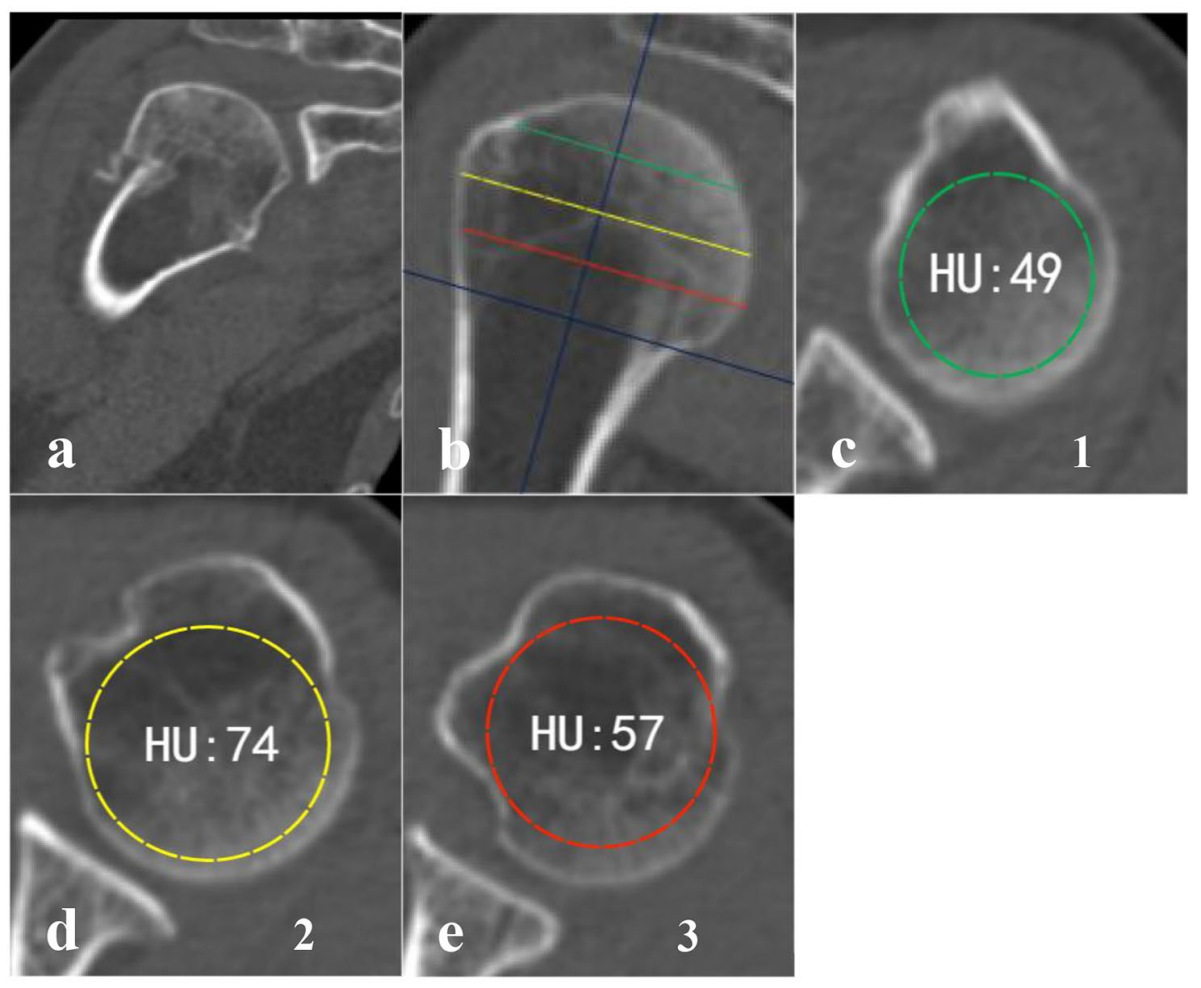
Method of measuring the proximal humerus average HU value. CT image of the patient with proximal humerus fracture(a). CT image of the same patient, the contralateral uninjured proximal humerus. The center of the humerus medullary cavity and the height of the head were determined first. Then, three axial planes equidistant to each other were defined(b). ROIs were placed in the humerus head in each of the previously defined axial planes(c-e). CT, computed tomography; ROI, region of interest
